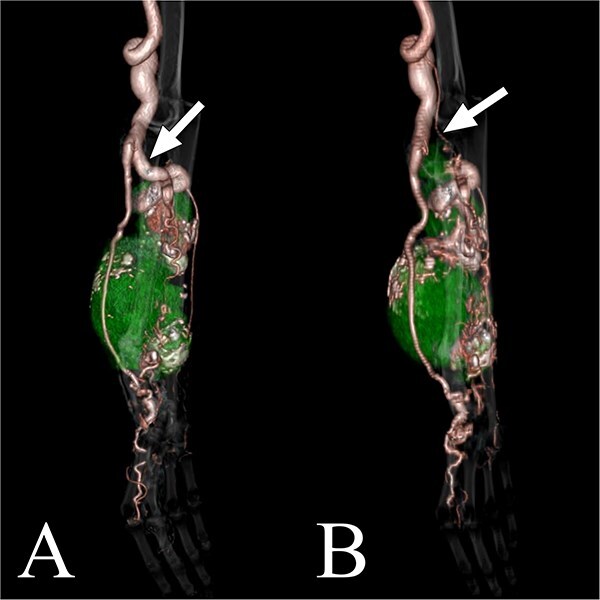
Three-dimensional CT angiography before and after proximal ulnar artery ligation. (A) Preoperative imaging demonstrating the ulnar artery aneurysm. The arrow indicates the ulnar artery at the site of ligation. (B) Imaging obtained 7 months postoperatively. Despite proximal ligation, the aneurysm persisted, and the arrow indicates a newly developed collateral vessel supplying the aneurysm.
